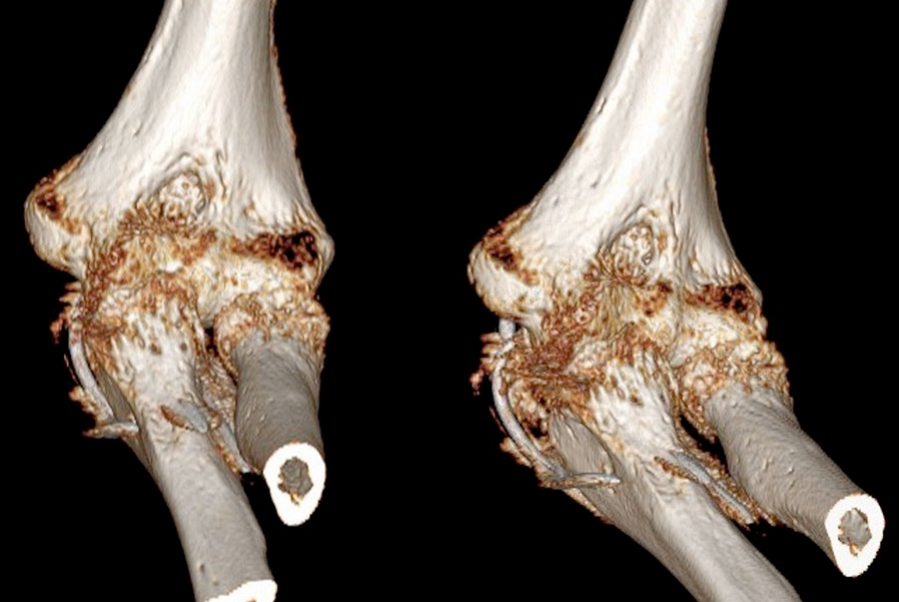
Malpositioned K wires after olecranon TBW. 3D computed tomography scans show the K wire placement within the radioulnar space at the radial tuberosity 6 weeks after initial surgery
